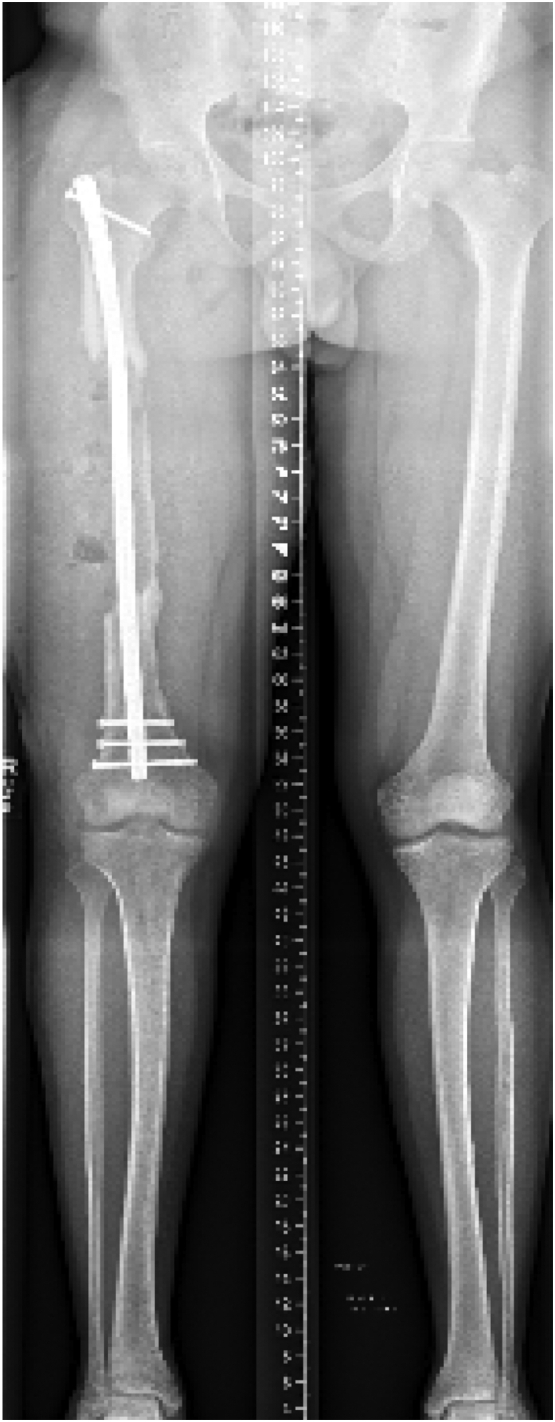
Standing alignment radiograph taken two weeks after second stage of his Masquelet operation, demonstrating intramedullary nail in place, with 20 cm defect with bone graft in place.
